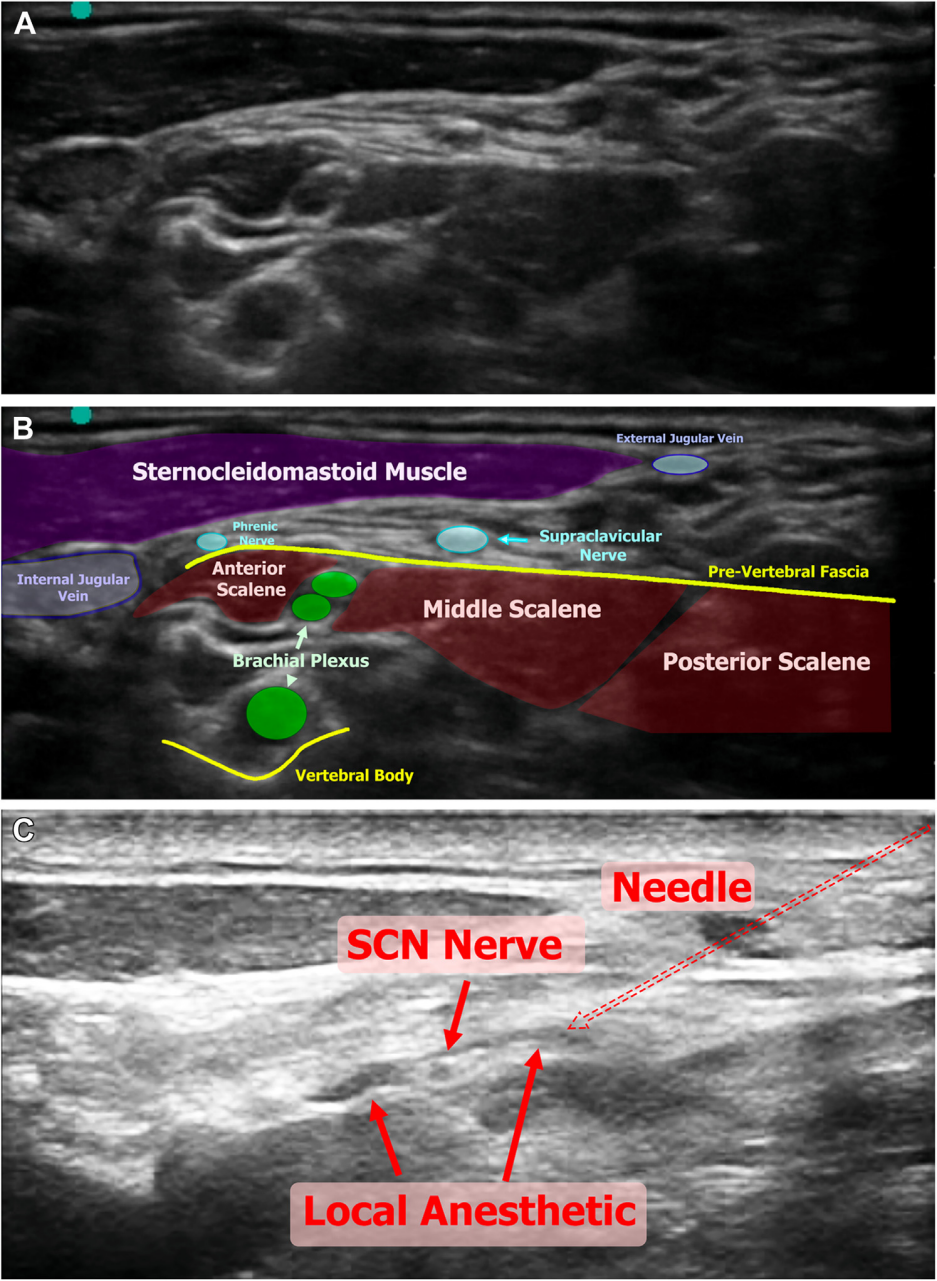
Ultrasound-Guided PECS 1 Block (A) The probe is positioned 2 inches below the clavicle in parallel orientation to the clavicle. The probe is then moved until the thoracic branch of the thoracoacromial artery is in view, which separates the pectoralis major and minor muscles. (B) Same anatomy with a labeled overlay. (C) Injection of the local anesthetic into the fascia between the pectoralis major and minor muscles taking care not to injure the blood vessels. PEC = pectoral nerve; SCN = supraclavicular nerve.
